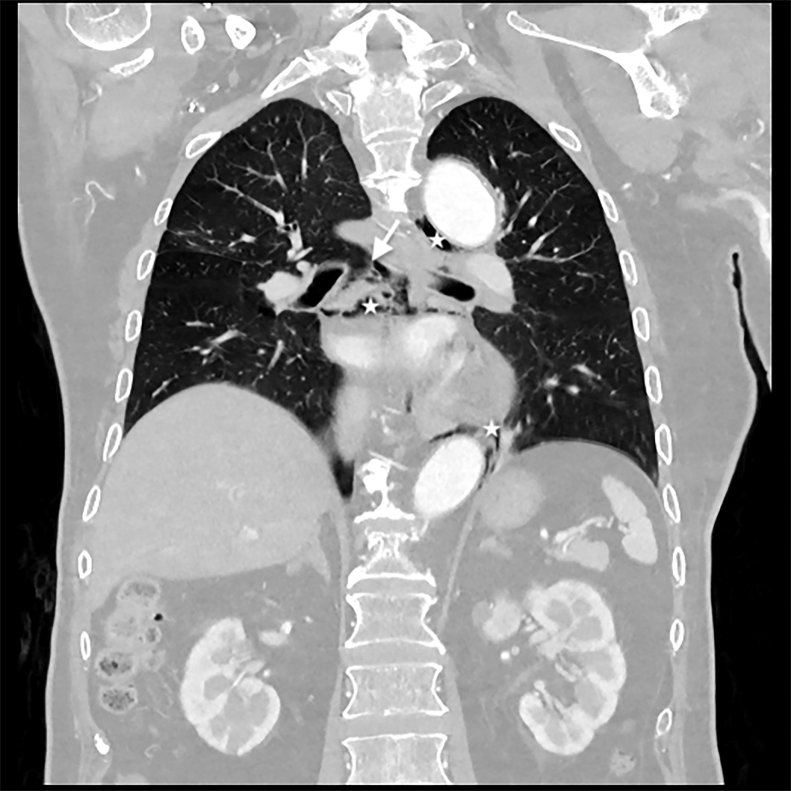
Coronal reformatted contrast-enhanced chest computed tomography demonstrates tracheomediastinal fistula (arrow) at the level of the carina with extensive pneumomediastinum and pneumopericardium (star) secondary to left upper lobe lung malignancy.
